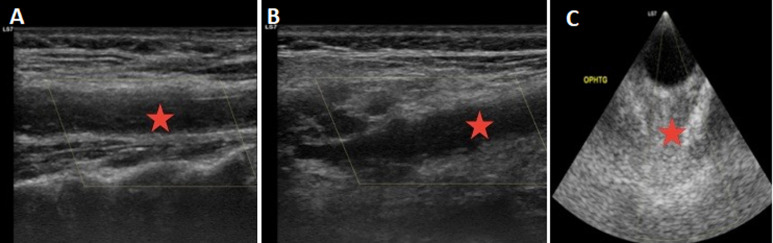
echo-doppler of the supra-aortic vessels (A, B): endoluminal material at the level of the left common carotid artery extended to the carotid bulb with no color doppler plug; C) absence of color doppler plug in the left ophthalmic artery
